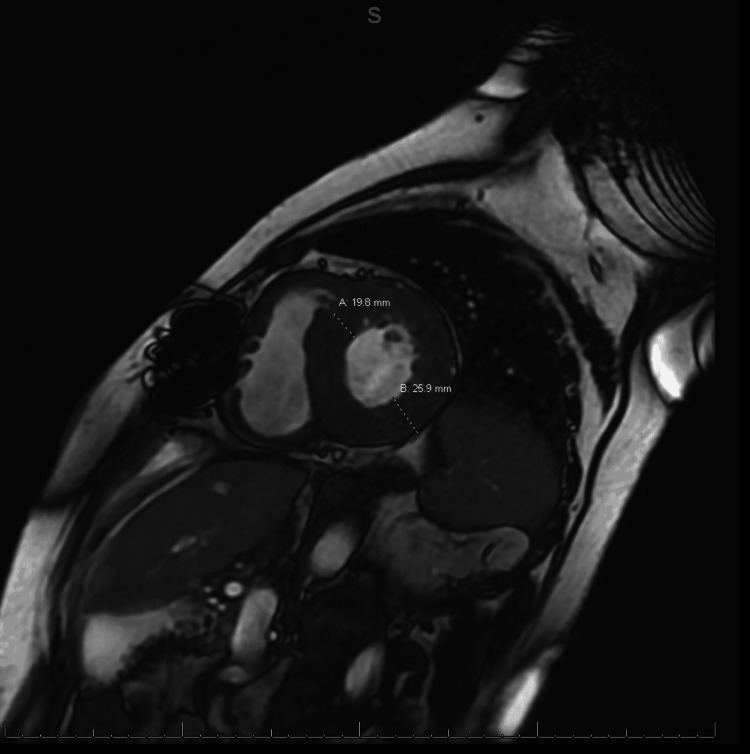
Cardiac MRI showing anteroseptal wall thickness of 19.8 mm and posterior lateral wall thickness of 25.9 mm.
